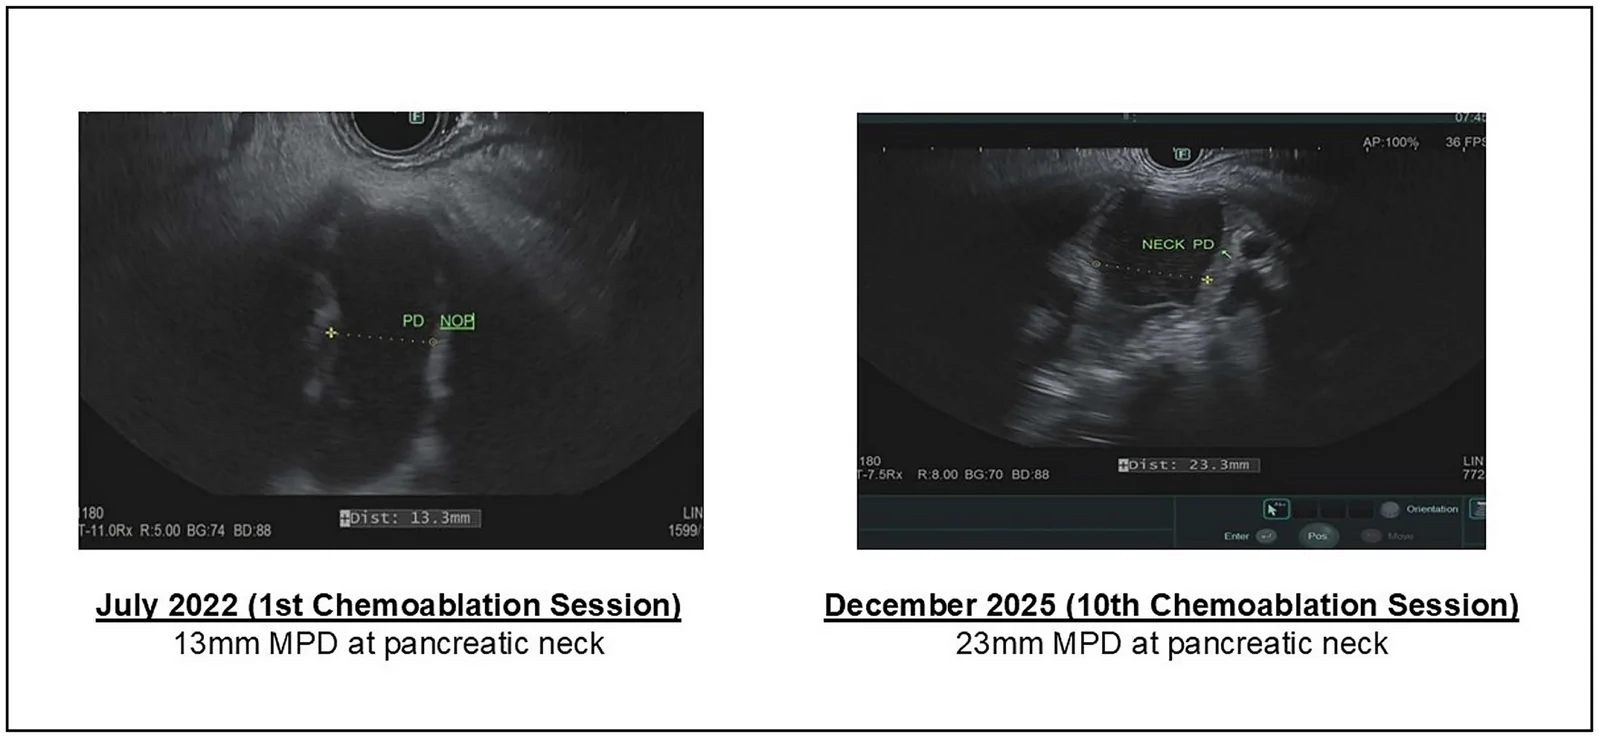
Endoscopic ultrasound imaging of the patient’s main pancreatic duct at the level of the pancreatic neck.
